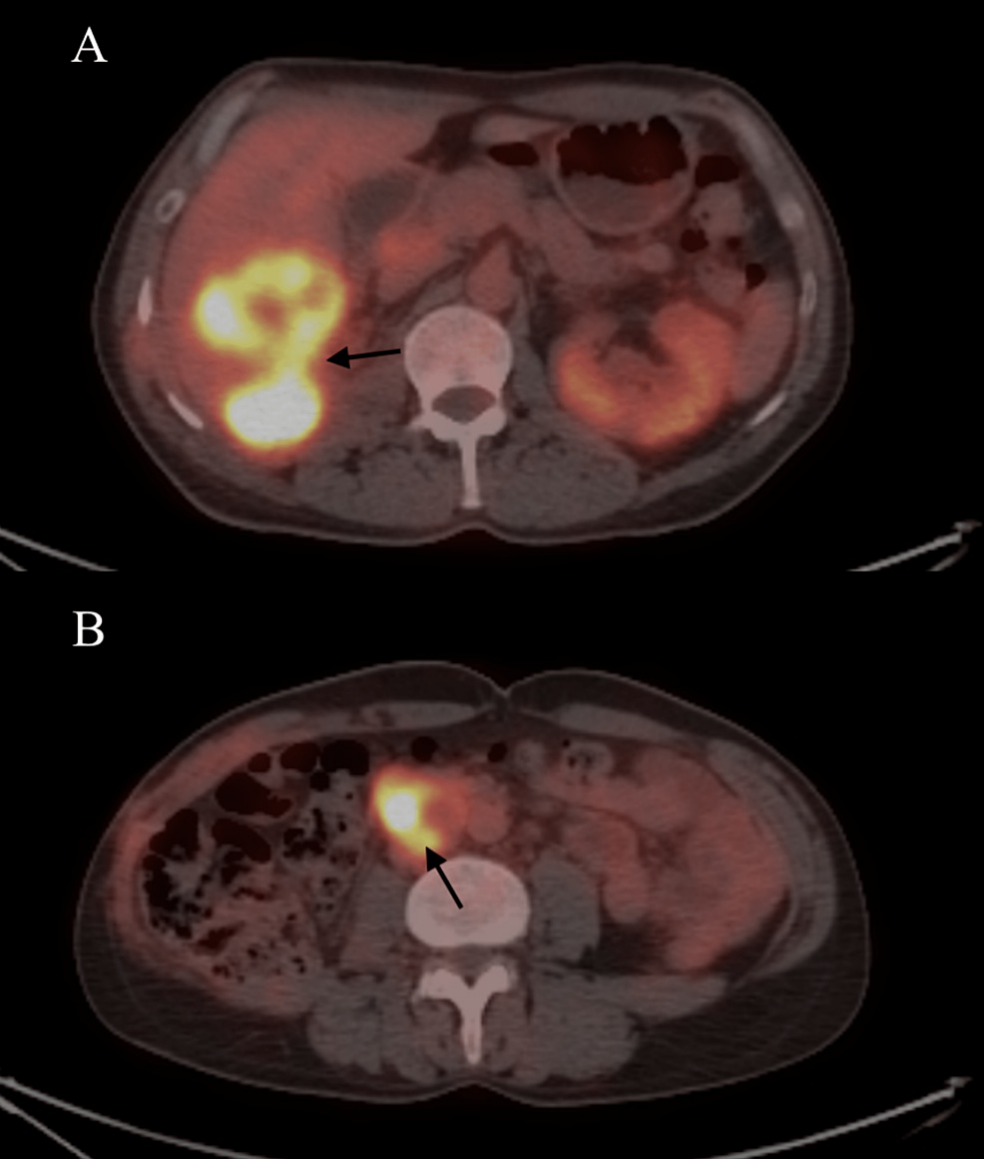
FDG-PET CT sections during follow-ups showing a metastatic mass in the liver (A) and metastatic lymph nodes in the aortocaval region (B) with arrows.
